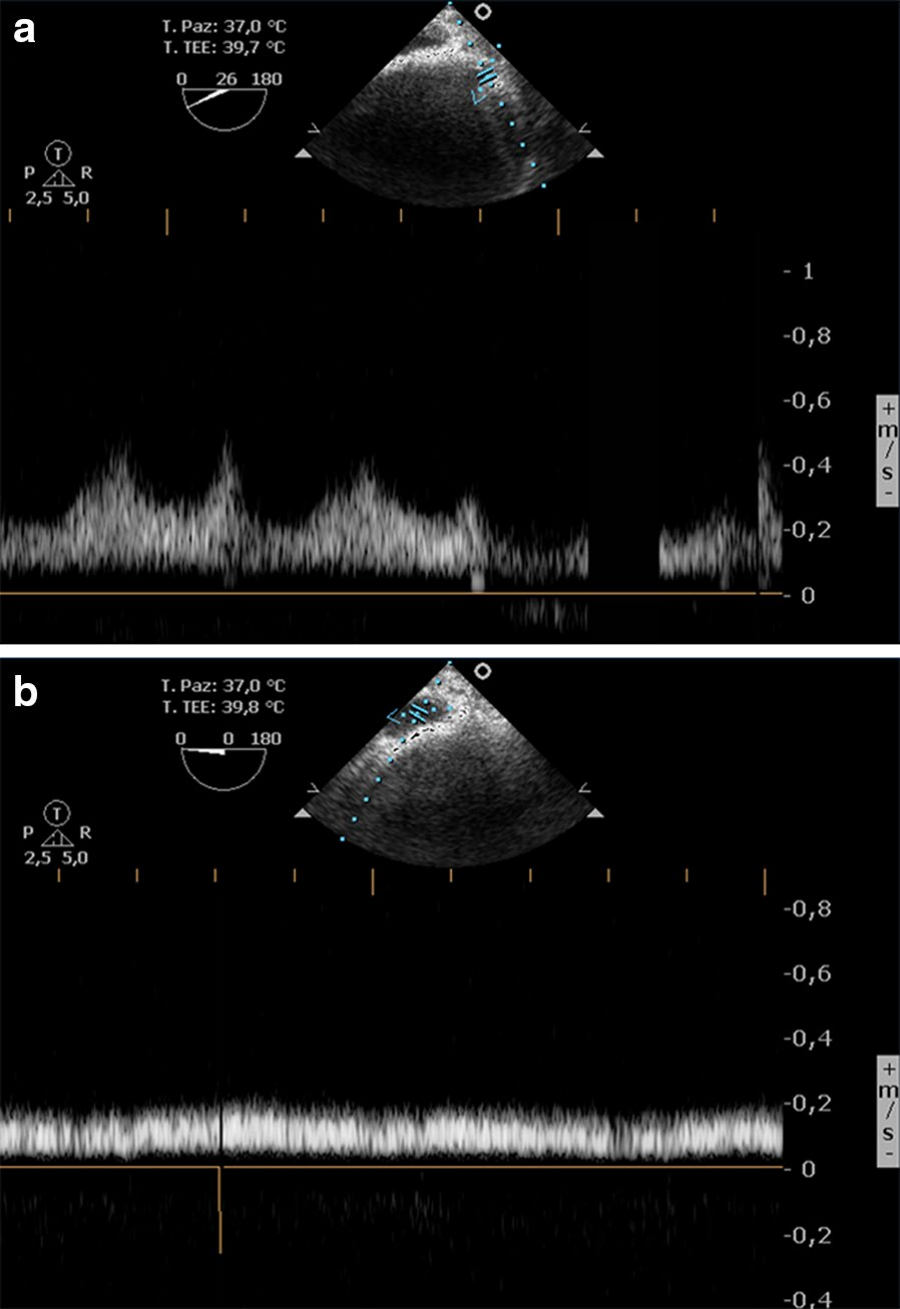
Pulse Wave Doppler (PW) analysis, measuring adequate blood flow velocity, in the suprahepatic vein (a) and inferior vena cava (b) after the patch repair
